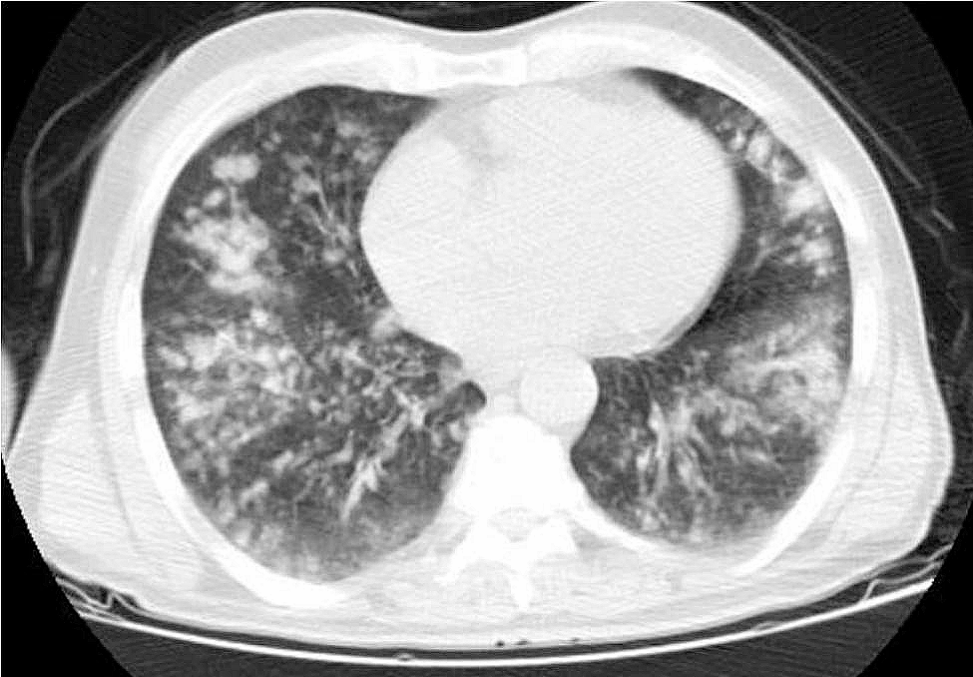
Lung CT scan of a 71-year-old man with concomitant TB. Centrilobular nodules and lobular consolidation in both lungs
